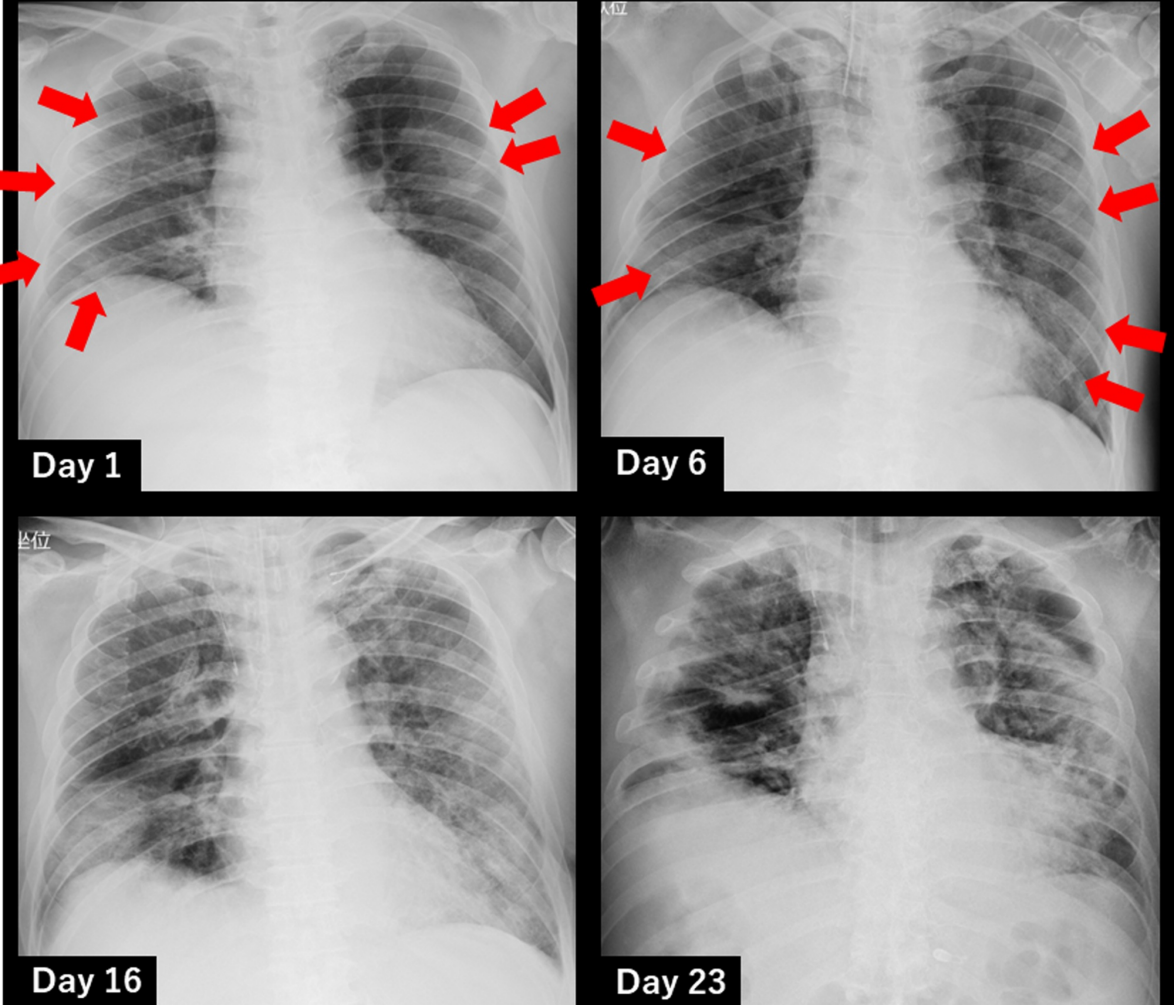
Changes in chest X-ray images
